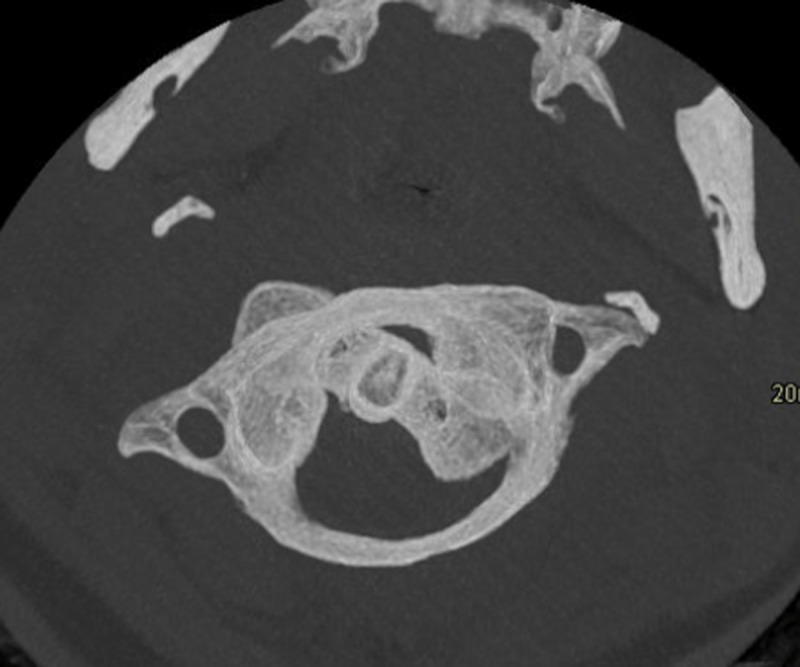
Atlas and axis rotate as one unit on CT with maximal contralateral head rotation.
